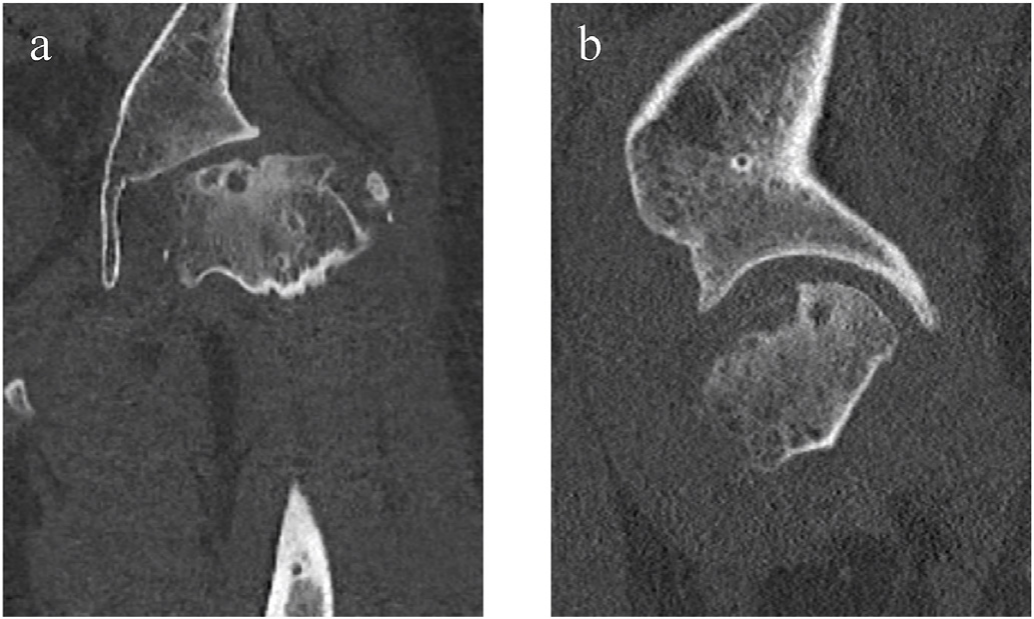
Computed tomography, a) Coronal view, b) Sagittal view at final follow-up.
